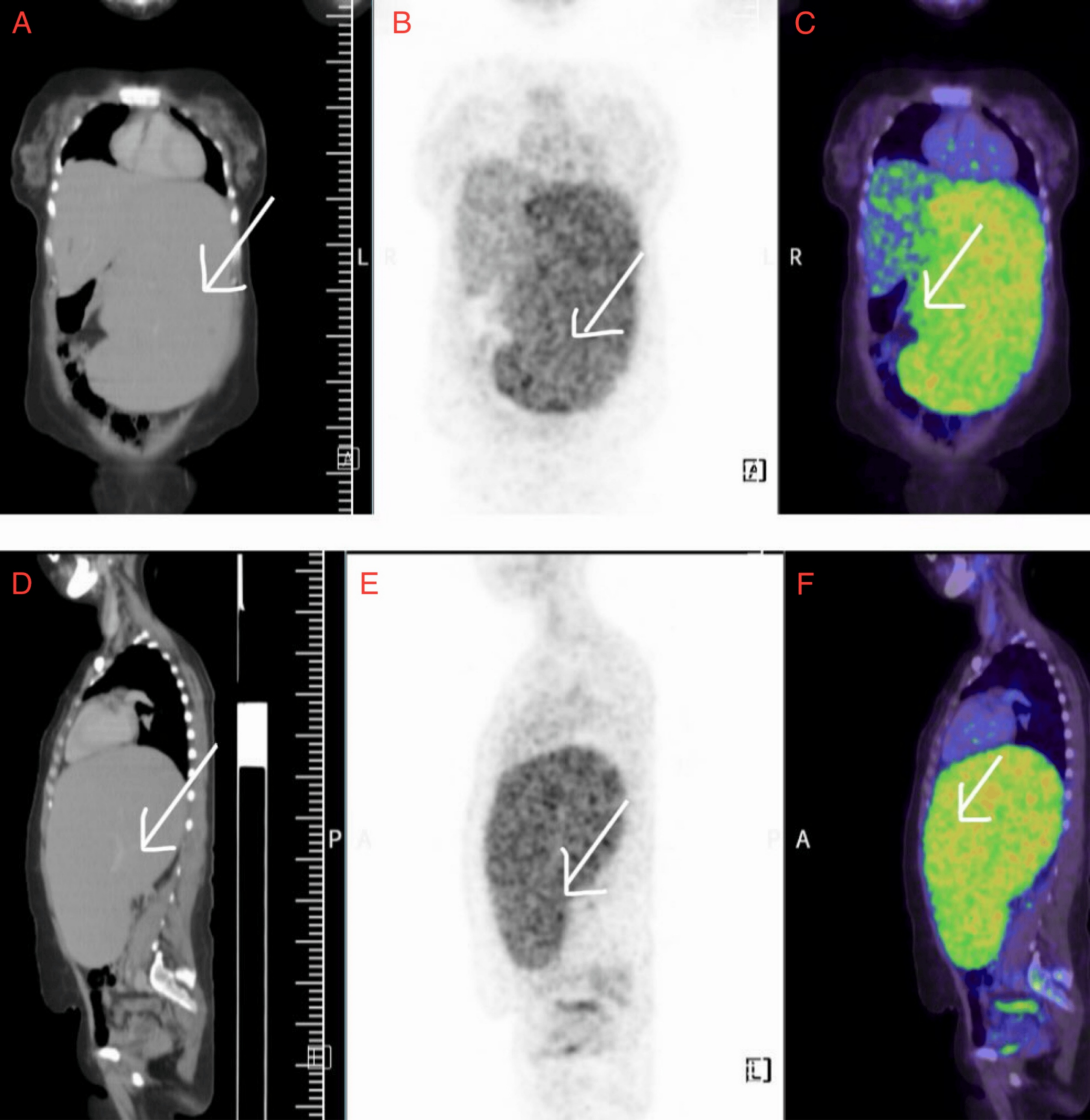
18F-FDG PET-CT revealing isolated splenomegaly without lymphadenopathy Spleen appears enlarged (splenic index-4775), crossing the midline with increased metabolic activity (SUVmax 4.12) (A) Coronal CT shows massive splenomegaly crossing midline. (B) Coronal PET demonstrates diffuse increased FDG uptake within the spleen. (C) Coronal fused PET-CT confirms metabolically active splenic enlargement (SUVmax 4.12). (D) Sagittal CT reveals marked craniocaudal splenic enlargement (splenic index ~ 4775). (E) Sagittal PET shows diffuse splenic FDG uptake. (F) Sagittal fused PET-CT confirms isolated splenic involvement with no evidence of nodal or extra-splenic disease FDG: fluorodeoxyglucose; PET: positron emission tomography; CT: computed tomography; SUVmax: highest standardized uptake value
